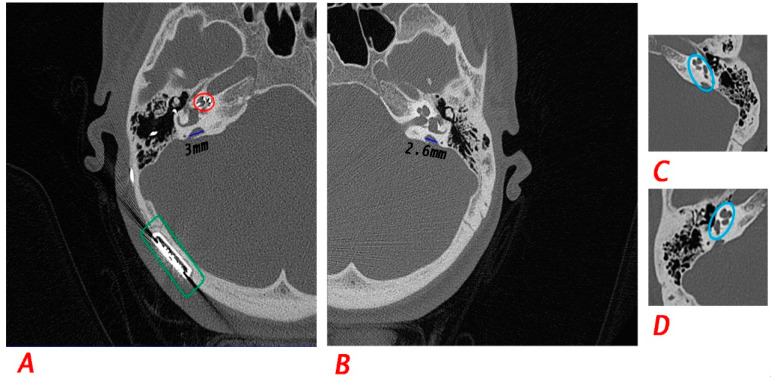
Temporal CT scan findings for F1: IV-3 and F1: IV-4. (A,B) Axial view of bilateral temporal bone CT scan for F1: IV-4 at the age of 17 years old post cochlear implantation where the blue lines represent the length of the EVA. The red circle shows the electrodes of the cochlear implant and the green rectangle shows the implanted cochlear device (A) for the right side and (B) for the left side. (B,C) Axial view of bilateral temporal bone CT scan for F1: IV-3 at the age of 25 years old showing features of incomplete partition II (Mondini Deformity) indicated by the blue circle (C) for the left side and (D) for the right side.
